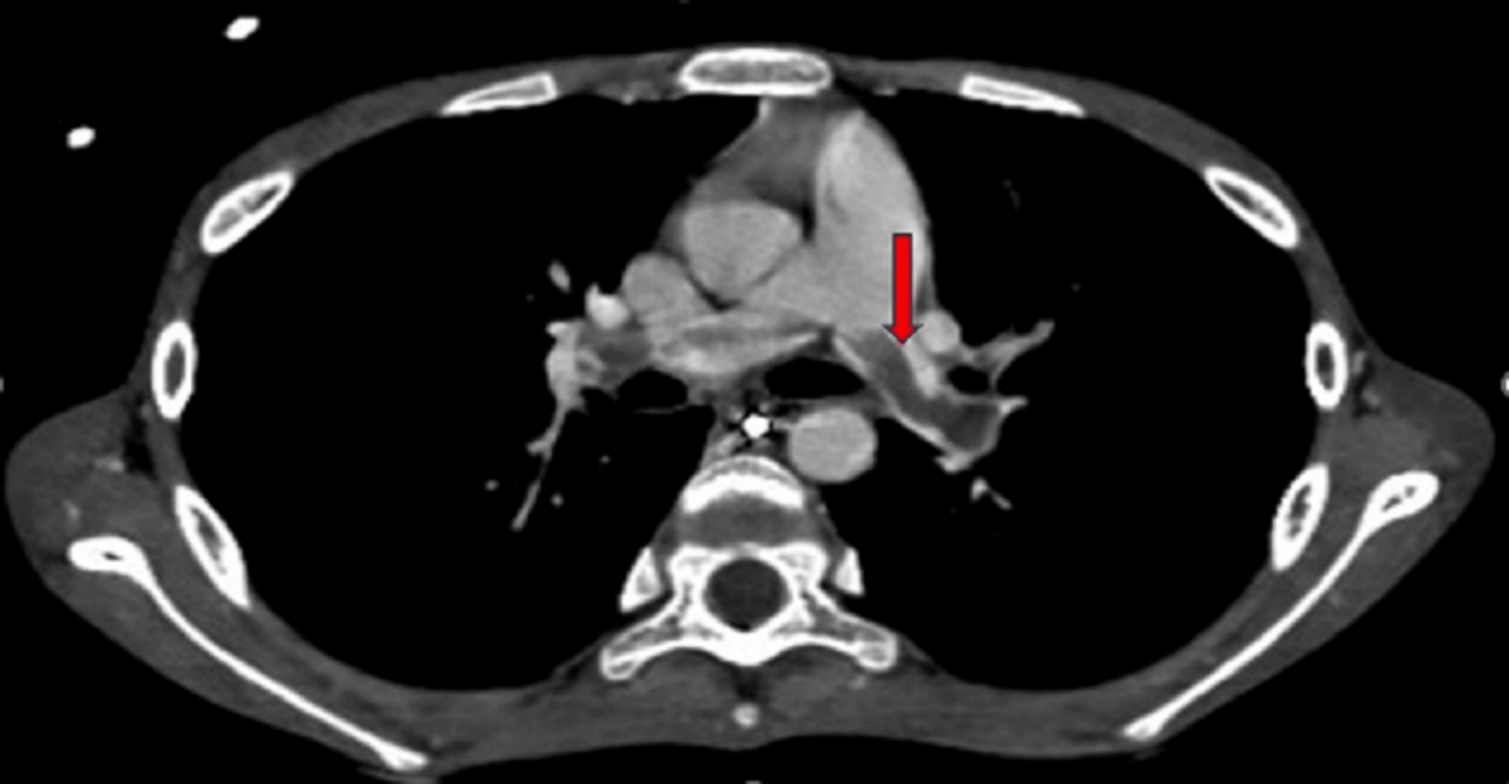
Abdominal CT scan showing saddle embolism in pulmonary artery and its branches (arrow) CT: computed tomography
